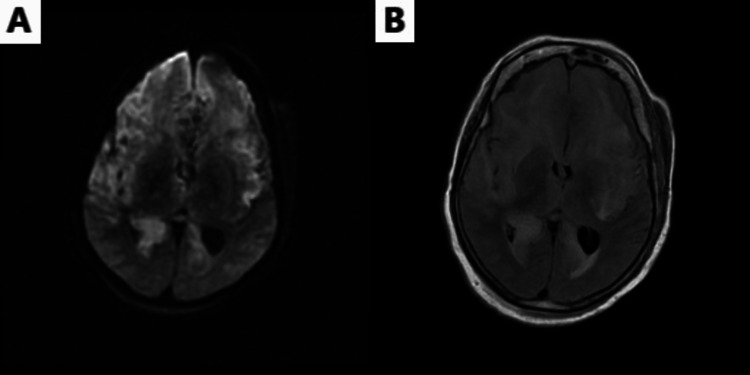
(A) Day 13: diffusion-weighted MRI showing progressive restricted diffusion bilaterally; (B) day 13: MRI T2 imaging showing advanced edematous change involving the bilateral mesial frontal lobes, insular lobes, external capsules, medial temporal lobes, and anterior temporal lobes. MRI: magnetic resonance imaging; T2: transverse relaxation time.
